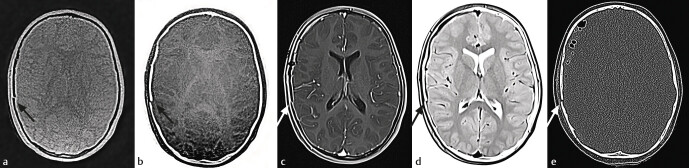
Comparison of ZTE vs. VIBE vs. CT for detection of skull fracture.
